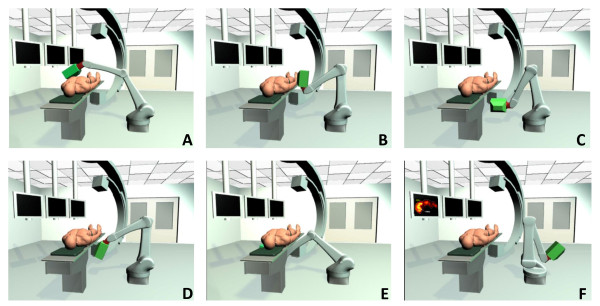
Example of a multi-pinhole SPECT implementation in a catheterization room using a six-axis arm robot. (A, B) The orbit motion above the patient. (C) The end-stage rotation drive of the robot rotates the detector around itself to turn the collimator side upward. (D, E) The orbit motion under the patient. (F) In home position, the system leaves the space around the patient free. During the orbit motion, the robot rotates slowly on its pedestal to provide a helical acquisition. See animation in Additional file 1.
